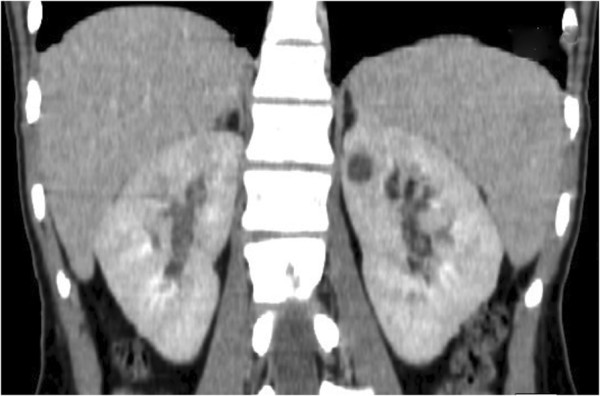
Coronal multiplanar reconstruction (MPR) of the abdominal CT scan revealed a hypodense lesion in the upper pole of the left kidney.
